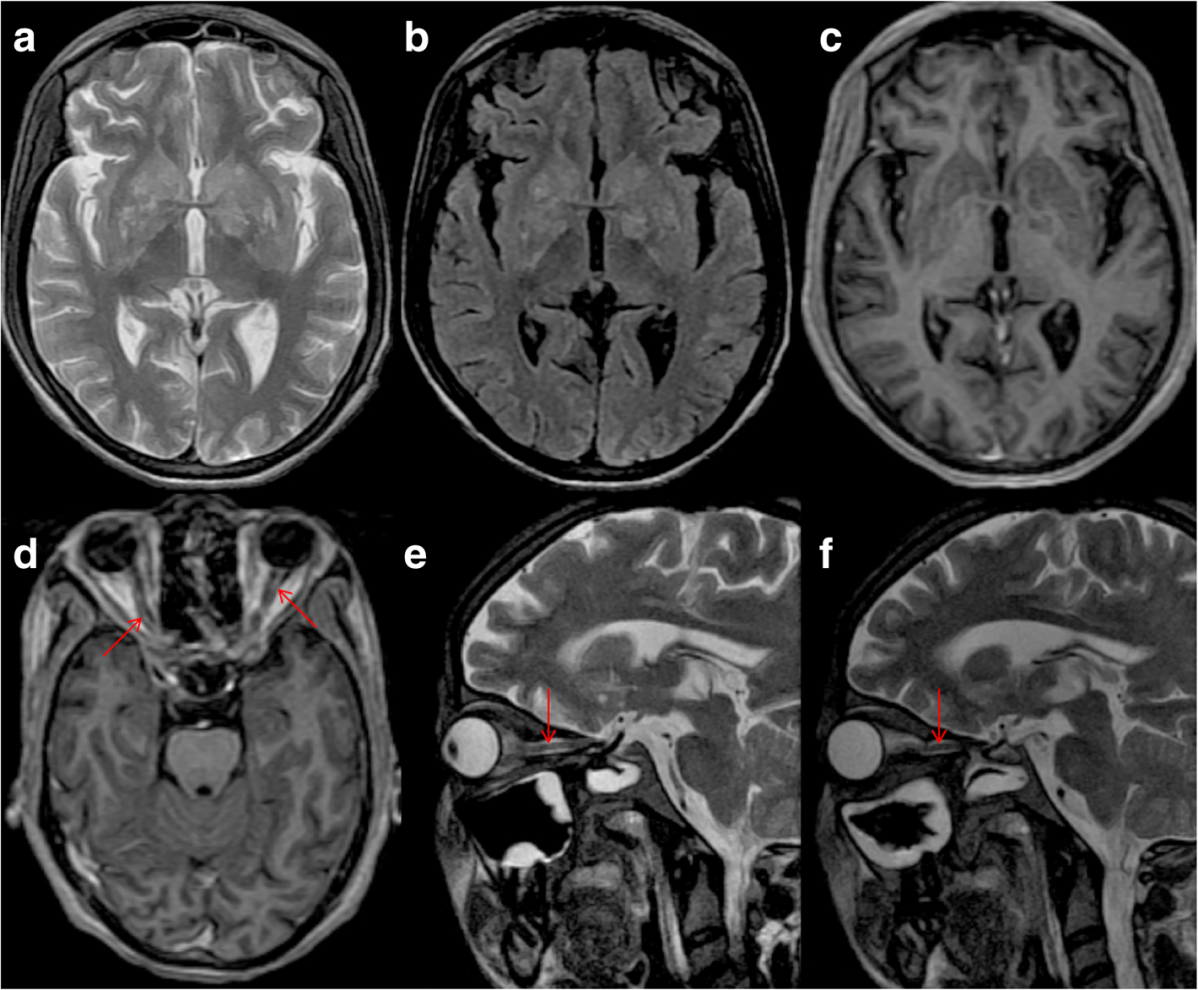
MRI of the brain depicts hyperintense signal intensity changes in the basal ganglia on axial T2-weighted (a) and FLAIR (b) sequences. There is no suggestion of contrast uptake in the basal ganglia (c) while both optic nerves demonstrate enhancement (d) on SPGR-GAD sequence. T2-weighted para-sagittal orbital sections show hyperintensity in the right (e) and the left (f) optic nerves (arrows)
